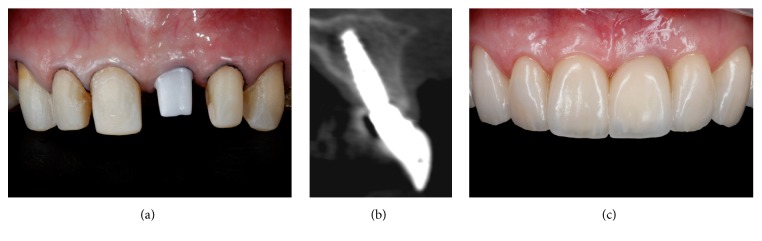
The adjacent teeth were prepared and an impression was made to produce all ceramic restorations (a). A CBCT demonstrated the creation of bone tissue around the implant and the increase of the gingival tissue one year after ITR (b). Results obtained one year after the zenith-driven rehabilitation (c).
